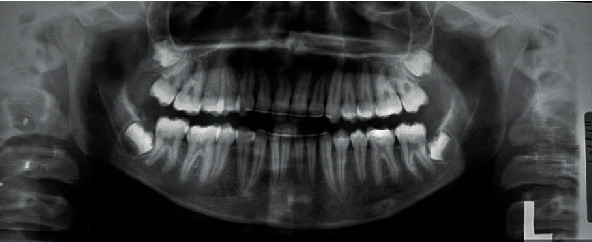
Posttreatment pantomogram X-ray.
